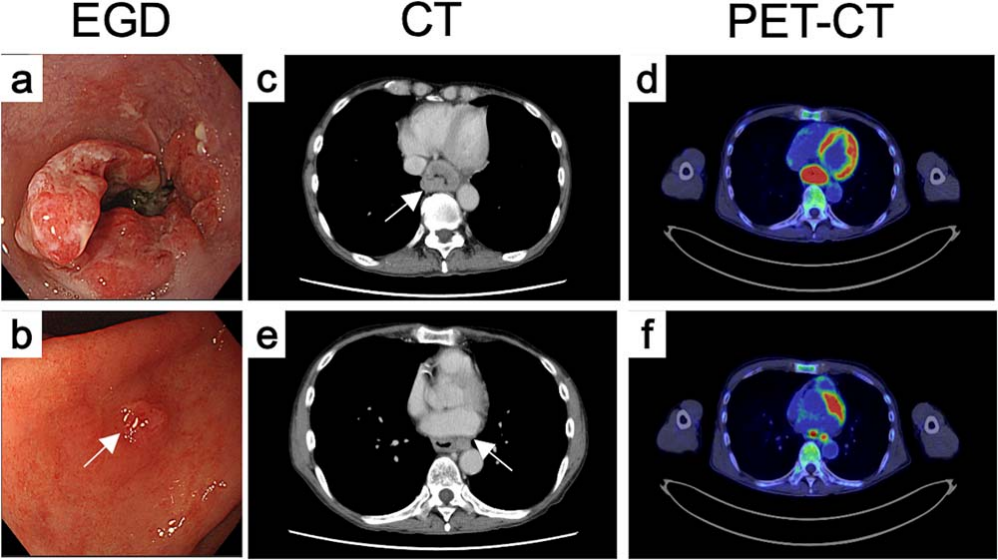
Endoscopic, CT, and FDG-PET findings before treatment. Endoscopic findings of (a) the primary tumor and (b) the gastric IM before treatment. (c) CT and (d) FDG-PET findings of primary tumor before treatment. (e) CT and (f) FDG-PET findings of metastatic LN.
